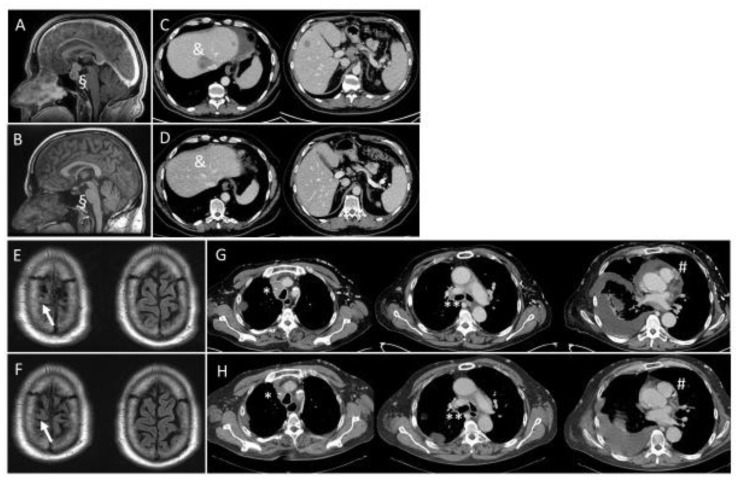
MRI and CT scans of two patients with *NTRK* fusion and Trk-directed therapy. Patient 1 (A–D) and patient 2 (E–H) showed responses to Trk-targeted therapy. (A,C,E,G) show MRI and CT scans at baseline. (B,D) show follow-up at month 27 (patient 1) and (F,H) at month 6 (patient 2). Metastatic sites, (A–D): § sellar/suprasellar, & liver parenchyma, (E–H): ↑ right precentral gyrus, * mediastinal,  hilar, # pericardial effusion and cardiac metastasis.
